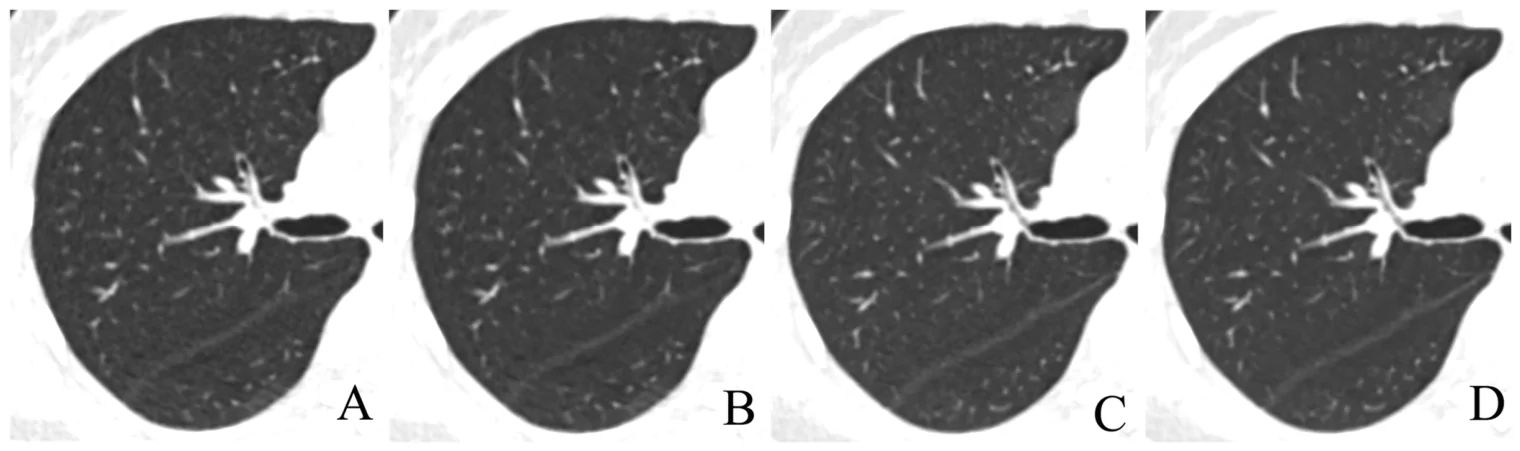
Representative axial lung-window CT images from one participant at the ULD and LD dose levels. Panels show (A) ULD-ADMIRE S3, (B) ULD-AiR, (C) LD-ADMIRE S3, and (D) LD-AiR images. All images are displayed using the same lung-window setting. The images were cropped and enlarged for display to facilitate visual comparison of image quality. ADMIRE—Advanced Modeled Iterative Reconstruction; AiR—AiR Denoising; CT—computed tomography; LD—low dose; ULD—ultra-low dose.
